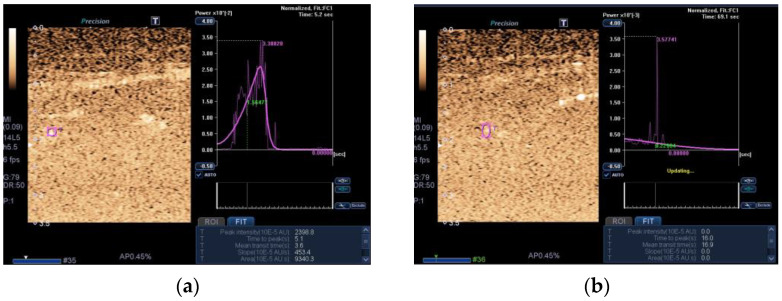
Transversal ultrasound of the QRF muscle after CEUS administration and time-intensity curve analysis in a 56-year-old patient with multiorgan failure shows (a) ROI (pink circle) in the area of maximum perfusion. (b) ROI (pink circle) in the area of minimum perfusion.
